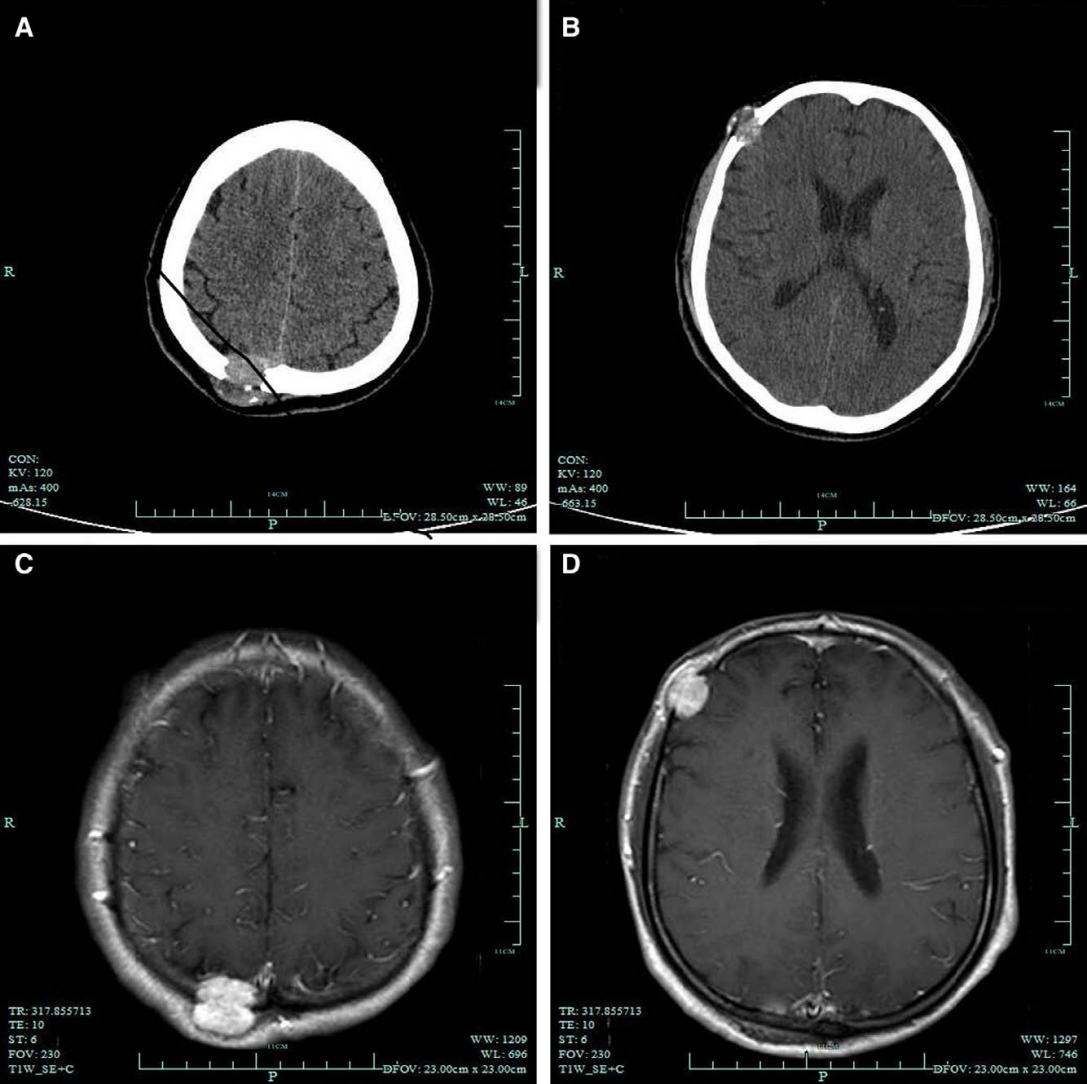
The detection results of CT and MRI. (A) CT scan showing right parietal bone destruction with soft tissue shadow. (B) Computed tomography scan showing right temporal bone destruction with soft tissue shadow. (C) T1-weighted image of the right parietal bone showing hyperintensity. (D) T2-weighted image of the right temporal bone showing hyperintensity.
